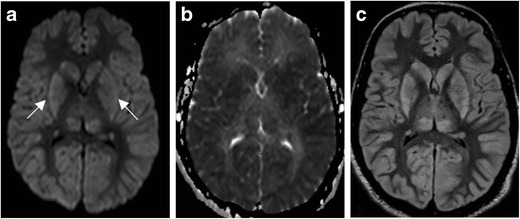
Hypoxic ischaemic encephalopathy. Sixty-eight-year-old man with prolonged cardiac arrest presenting with fixed and dilated pupils. DWI (a) demonstrates symmetric increased signal within the deep grey matter (*arrows*) and cerebral cortex. ADC (b) demonstrates corresponding low signal, consistent with reduced diffusivity. FLAIR (c) demonstrates corresponding symmetric increased signal throughout the deep grey matter and cerebral cortex
